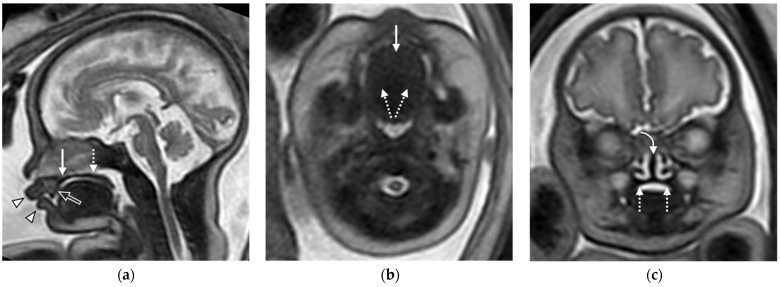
Fetal MRI of normal lip and palatal anatomy at 33rd week of gestation: (a) Sagittal T2-weighted image of the fetal head showing the intact upper and lower lips (arrowhead), primary palate (white arrow), secondary palate (dashed arrow), and alveolar ridge (open arrow); (b) axial T2-weighted image showing the intact primary palate (white arrow) fused with the bilateral secondary palates (dashed arrows); (c) coronal T2-weighted image showing the intact secondary palate (dashed arrows) fused with the nasal septum (curved arrow).
